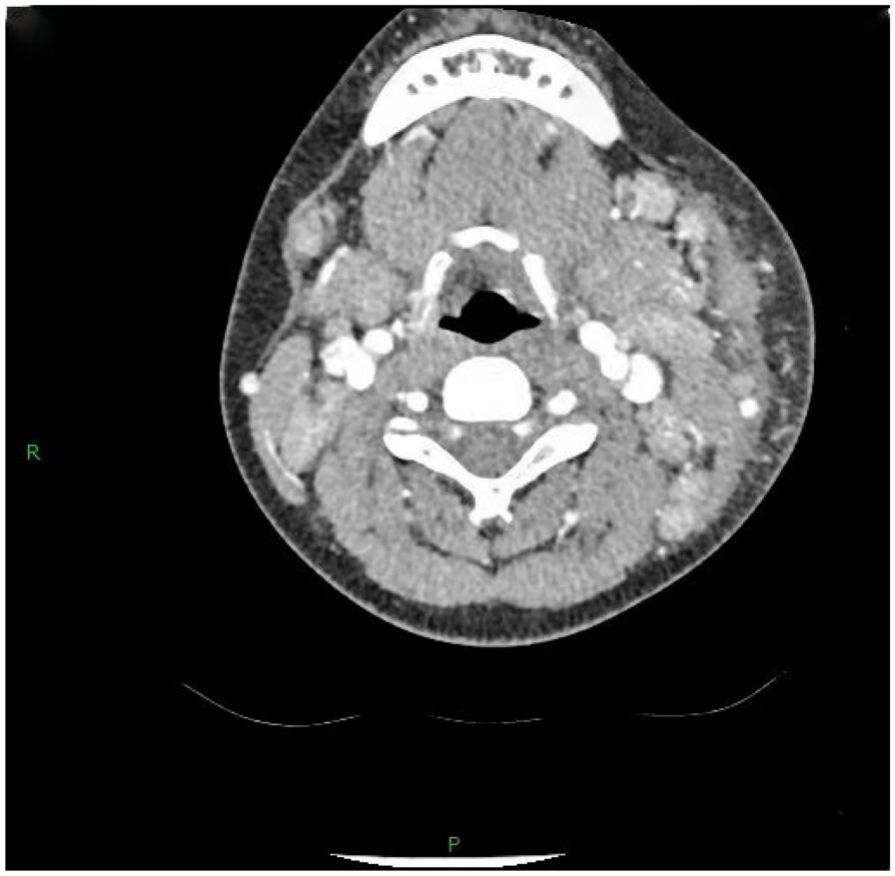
CT image of HNL lymph node.
